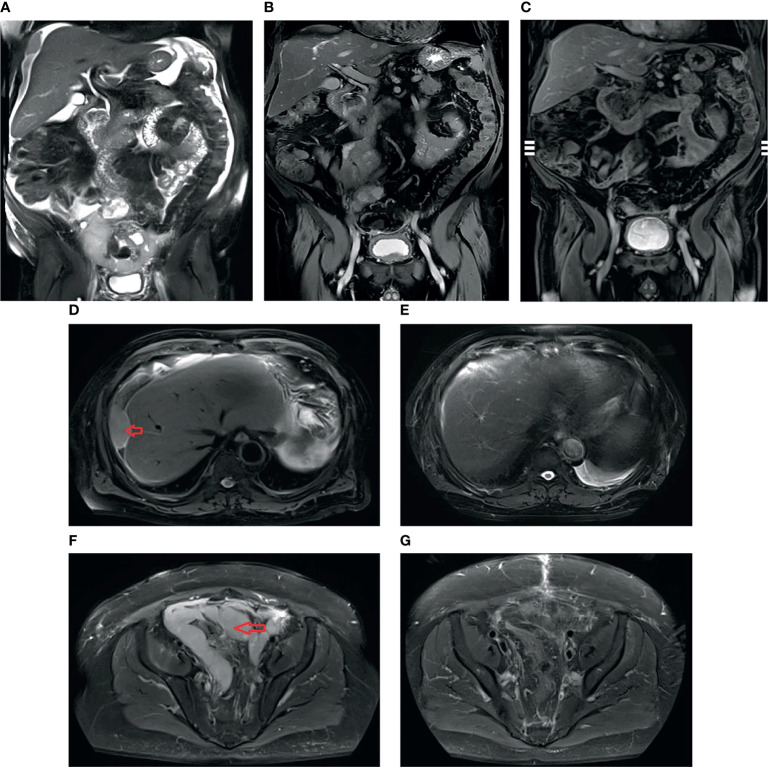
T2 sequence of coronal MRI: (A) before initial treatment with systemic chemotherapy (SC): massive ascites and the tumors; (B) after four cycles of SC: ascites disappeared, and the tumors became smaller and fewer in number; (C) after SC + cytoreductive surgery (CRS) + adjuvant chemotherapy treatment: the intra-abdominal tumor had resolved 3/4. T2 sequence of axial MRI: (D) before initial SC, arrow: peritoneal tumor; (E) after SC + CRS + adjuvant chemotherapy treatment, the tumor mass showed a complete response; (F) before initial SC, arrow shows “N”-shaped giant tumors; (G) after SC + CRS + adjuvant chemotherapy treatment, the tumors became smaller and fewer in number.
